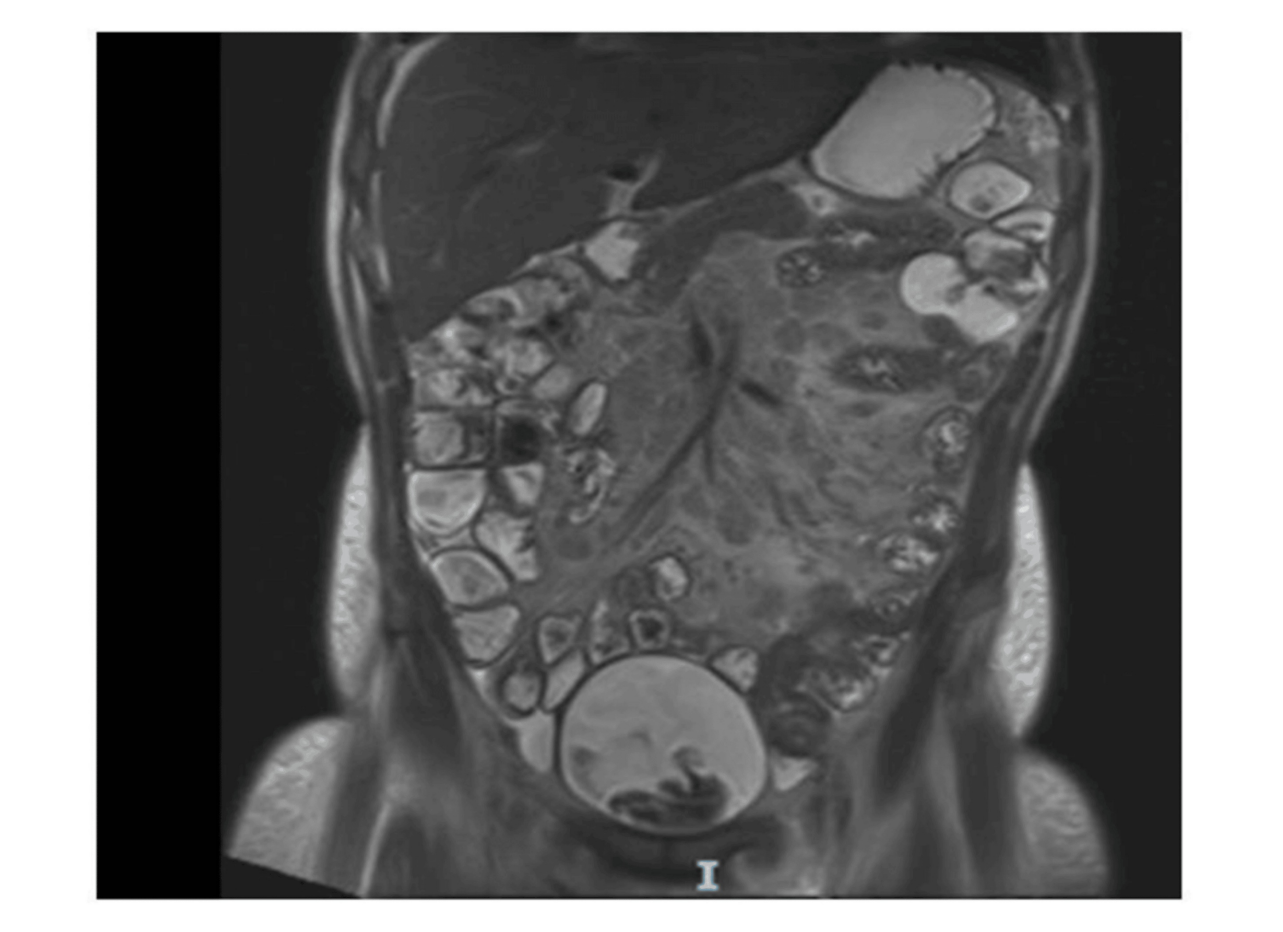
MRI image demonstrating a mesenteric nodal mass and extensive lymphadenopathy. MRI: Magnetic resonance imaging
